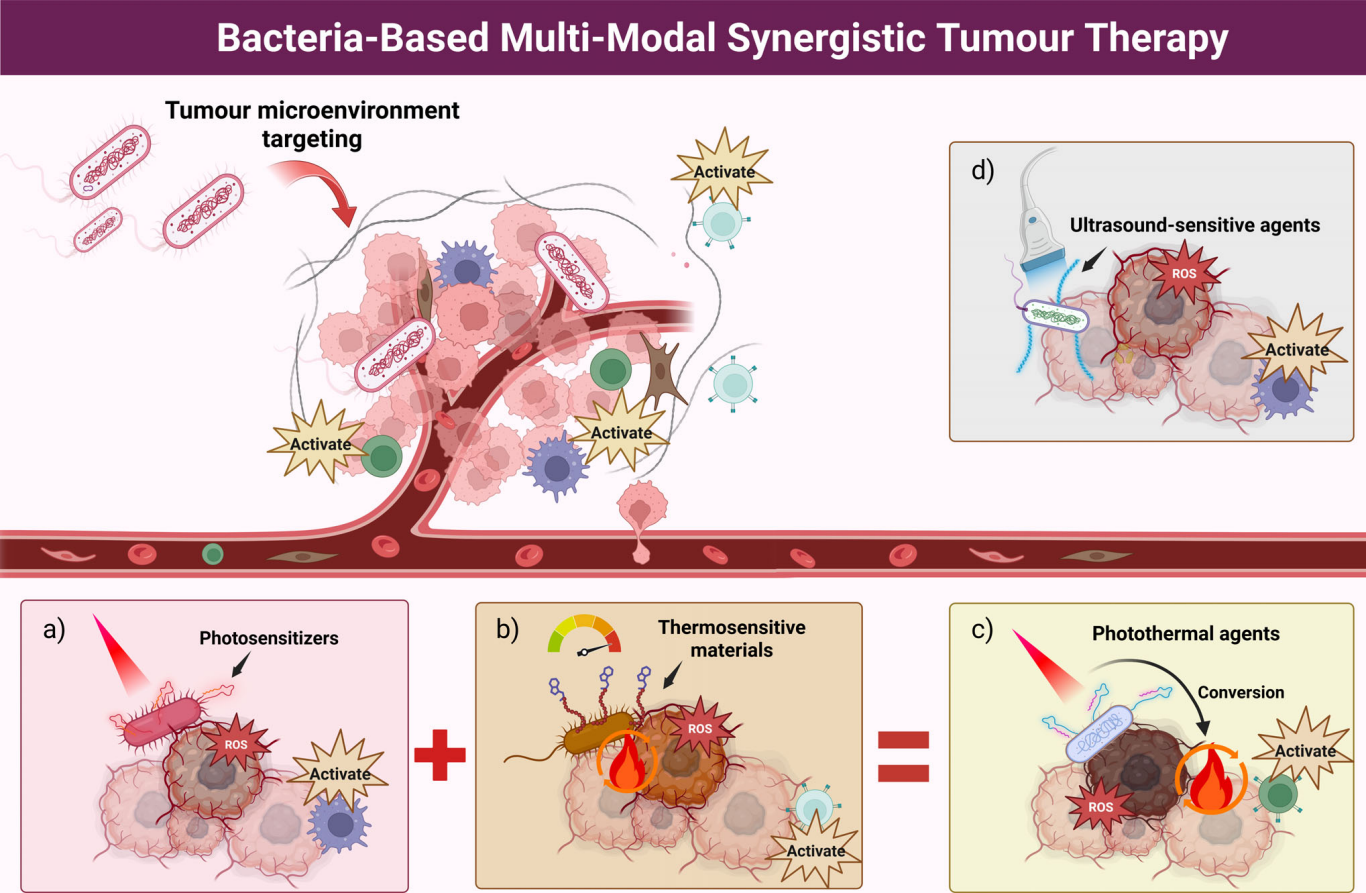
Bacteria can be used for multi‐effect synergistic cancer therapy. Bacteria themselves possess immune activation properties, which can stimulate the body's immune system to achieve tumour cell killing. (A) By using bacteria to deliver photosensitisers, immune and photodynamic therapy can be coordinated under stimulation by specific wavelengths. (B) Bacteria can be modified with thermosensitive materials, enabling immune and thermodynamic synergistic therapy through the control of local tumour temperature. (C) Combining bacteria with photothermal conversion materials allows for multi‐effect synergistic treatment, such as tumour photothermal therapy, maximising the tumour‐killing effect. (D) Bacteria can be utilised for the delivery of sonosensitisers or by constructing vibration‐sensitive bacteria, enabling immune and sonodynamic synergistic therapy under localised ultrasound stimulation. Created with https://www.biorender.com.
